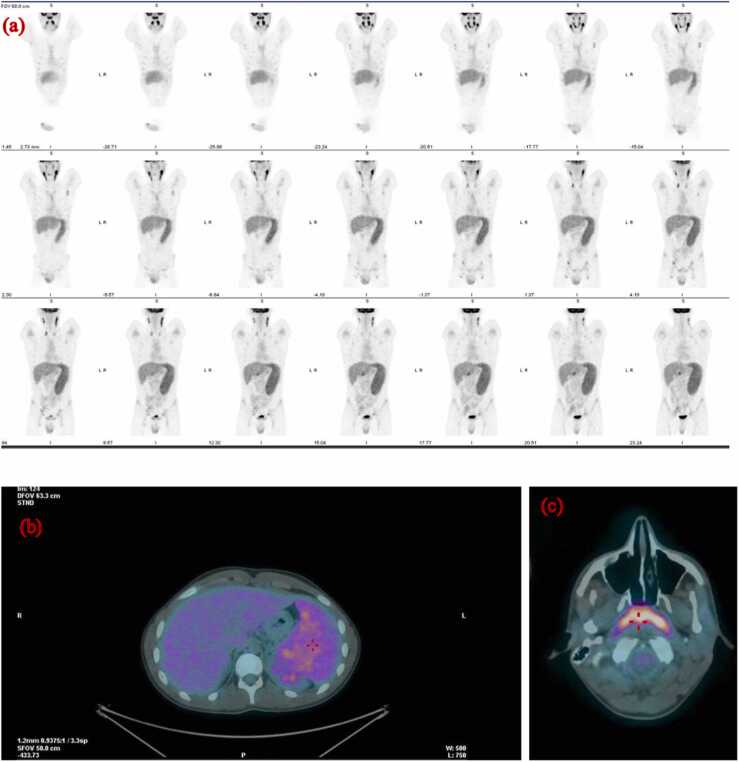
(a)^18^F-FDG PET/CT scan showed pathologically ^18^F-FDG uptake in enlarged lymph nodes in the bilateral regions of the neck, supraclavicular region, axilla, lung hili, perihepatic, paraaortic, para iliac, and groin. (b) A non-homogeneous distribution of ^18^F-FDG was in the spleen. (c) Pathologically ^18^F-FDG uptake was observed in the nasopharynx and oropharynx.
